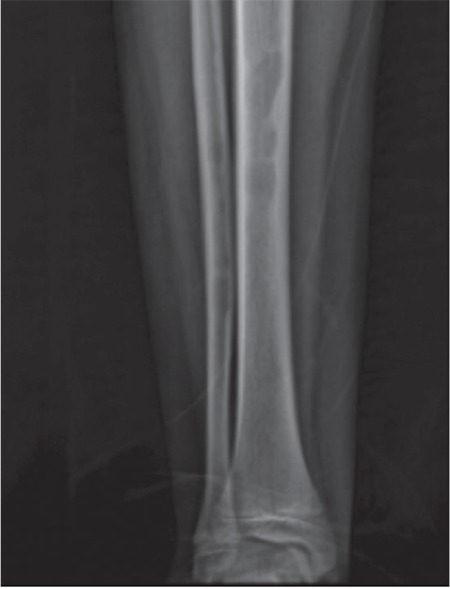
Direct radiography of the tibia.
